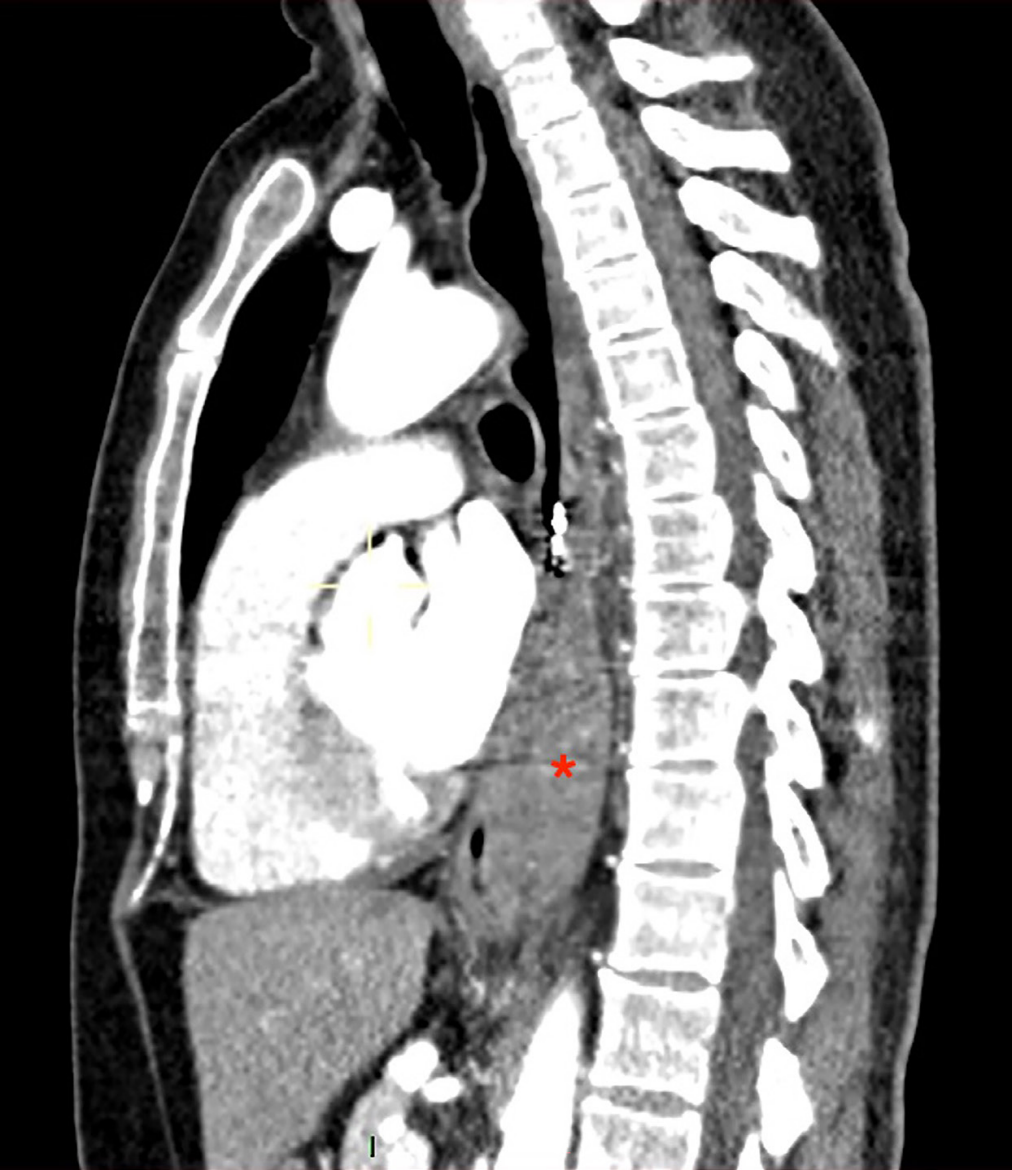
Contrast-enhanced, sagittal plane CT scan of the chest performed the 7th POD, at the time of the admission, showing the large submucosal esophageal haematoma which obliterates the esophageal lumen, without extraluminal leaks or evidence of intraluminal bleeding.
